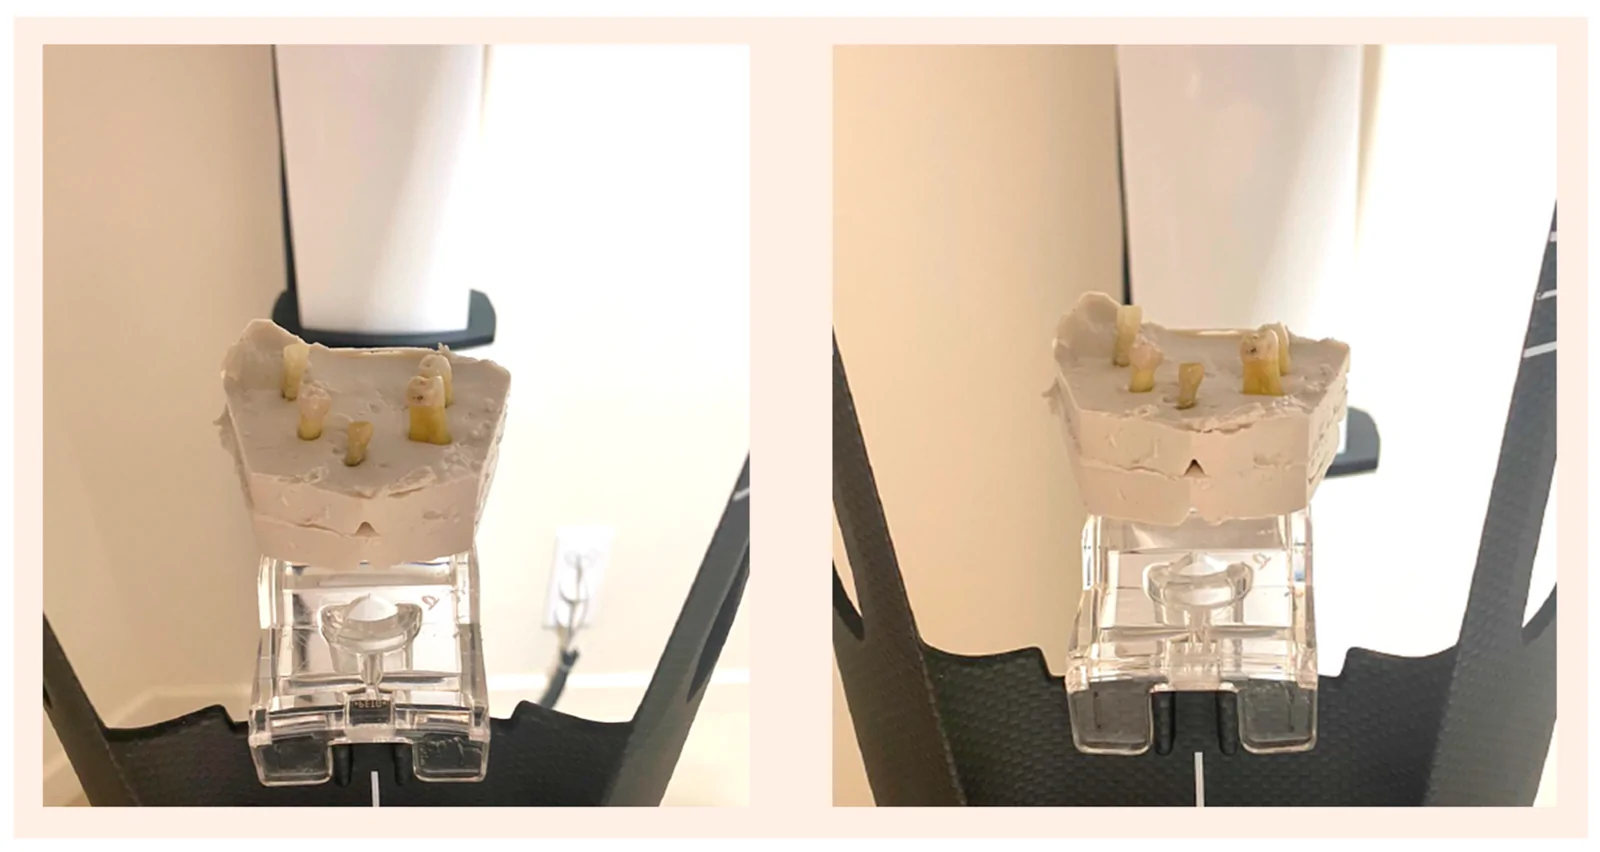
Conventional alginate-based holder positioned on the CBCT headrest.
